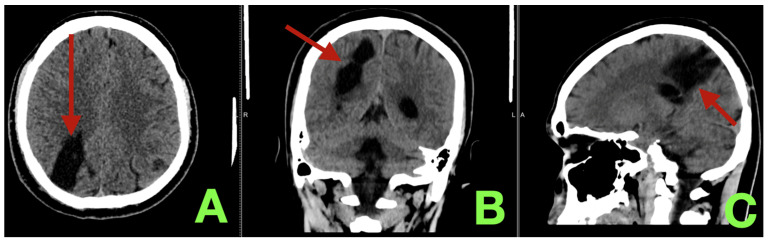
Three-month follow-up non-contrast CT. (A): Axial parenchymal window demonstrating a well-circumscribed right parieto-occipital encephalomalacic-gliotic cavity (arrow) corresponding to the healed hematoma–nidus corridor, with smooth margins, no residual hyperdensity, and no recurrent mass effect. (B): Coronal reconstruction confirming posterior convexity confinement of the resection bed (arrow), symmetric ventricular configuration, and absence of delayed hydrocephalus or periventricular injury. (C): Sagittal reconstruction showing superficial location of the mature resection cavity (arrow) without progressive edema, cystic expansion, or new hemorrhagic change, consistent with a stable, complication-free postoperative evolution and durable AVM excision.
